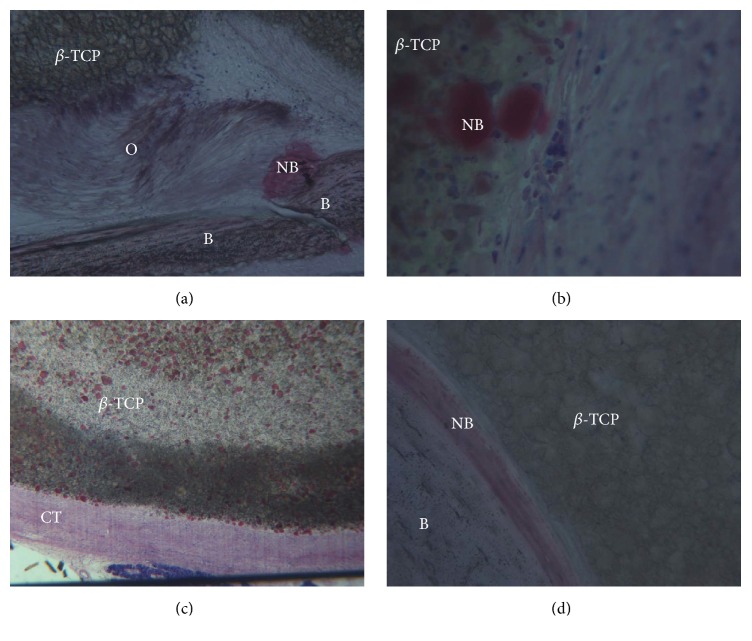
(a) *β*-TCP particles surrounded by osteoid matrix and new bone. Acid fuchsin and toluidine blue 100x. (b) An initial formation of immature bone (NB) extending from the periphery of the *β*-TCP is present. Lymphocytes were present around the *β*-TCP. Acid fuchsin and toluidine blue 200x. (c) *β*-TCP is completely surrounded by connective tissue (CT). No inflammatory cell infiltrate or macrophages are present. Acid fuchsin and toluidine blue 50x. (d) Only around some particles is it possible to observe the presence of new bone (NB) and mature bone. Acid fuchsin and toluidine blue 250x.
